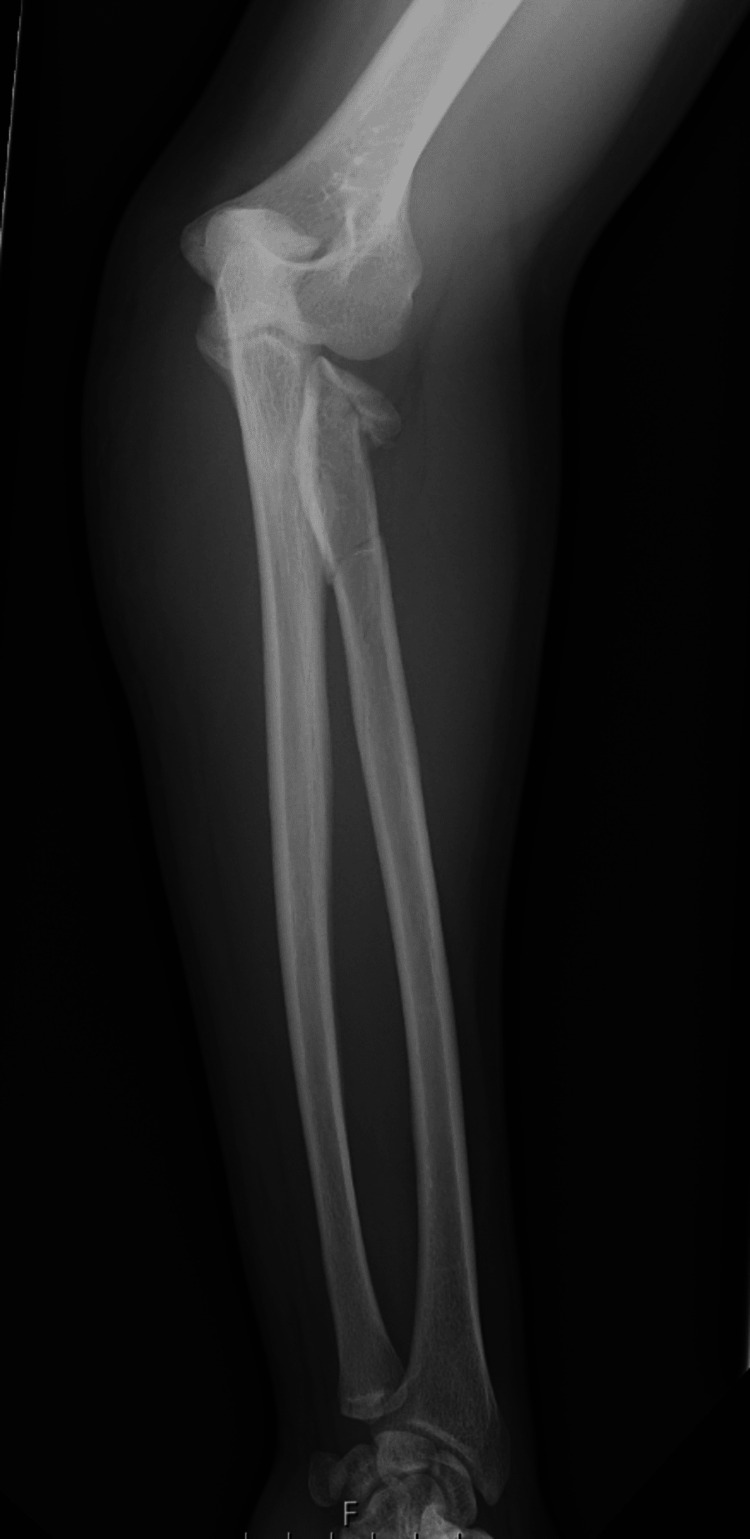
Preoperative radiograph confirming no distal radius/ulna fractures
